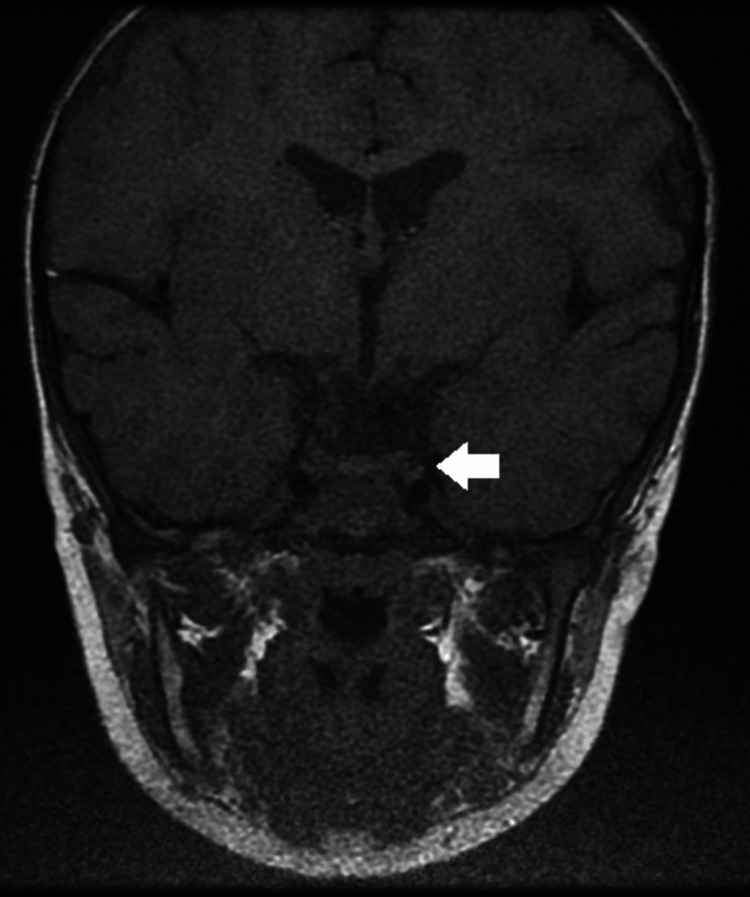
Brain MRI of the pituitary gland. Brain MRI demonstrated no evidence of pituitary hypoplasia or other structural abnormalities.
